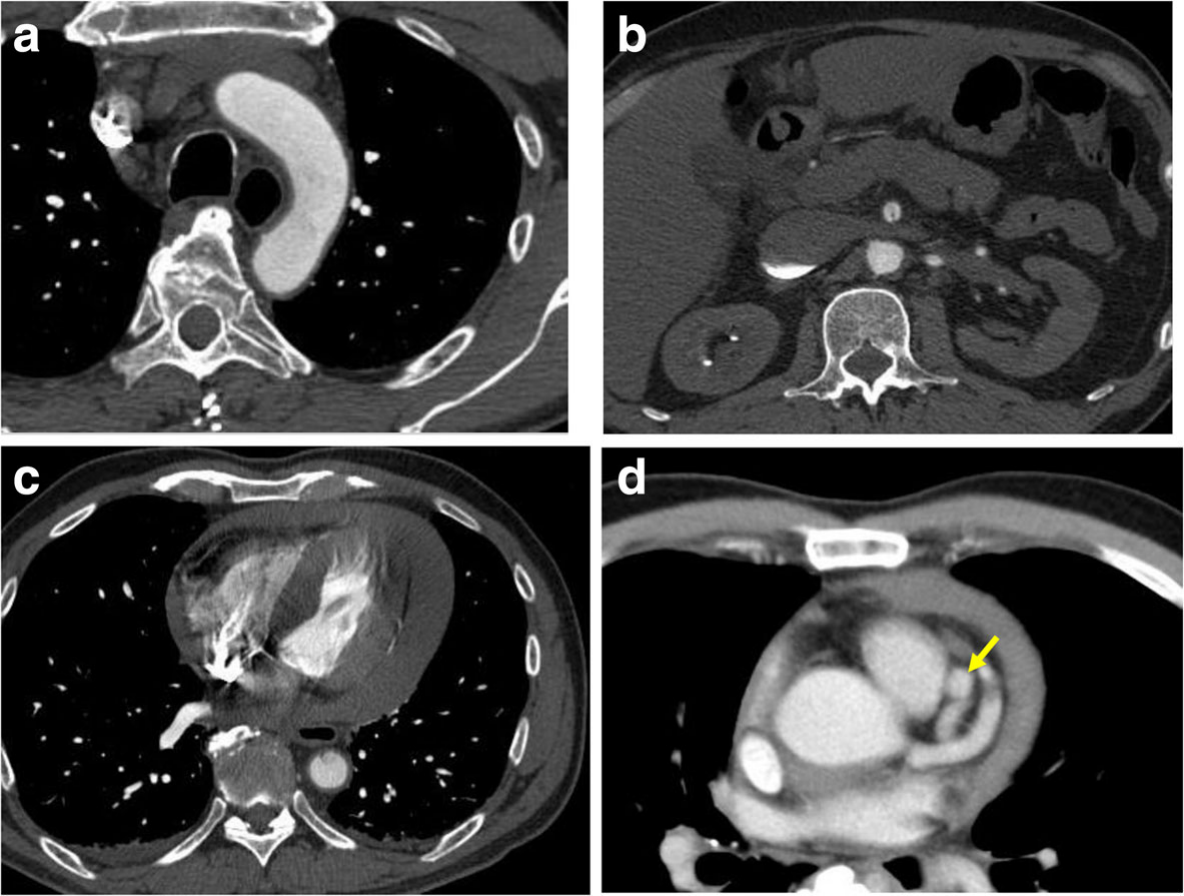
Preoperative images. Contrast-enhanced CT does not show a typical aortic dissection (a) and shows a dissection of the superior mesenteric artery (b). Cardiac tamponade was also revealed. c Coronary aneurysms appeared in a delayed phase (d)
